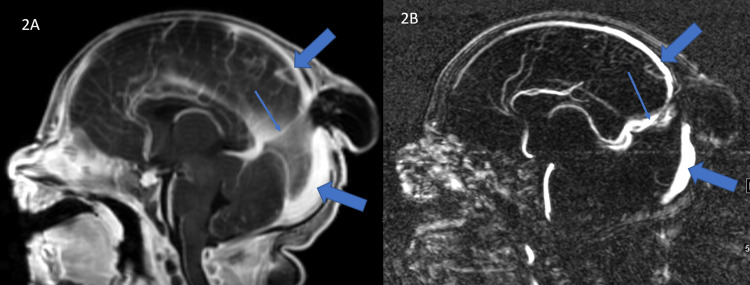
Persistent falcine sinus T1-weighted sagittal post contrast MRI image (A) and sagittal MR venogram image (B) show internal cerebral veins draining into the persistent falcine sinus (thin arrow) which drains into the superior sagittal sinus (thick arrow). The superior sagittal sinus is not seen in the midline at the site of encephalocele.
